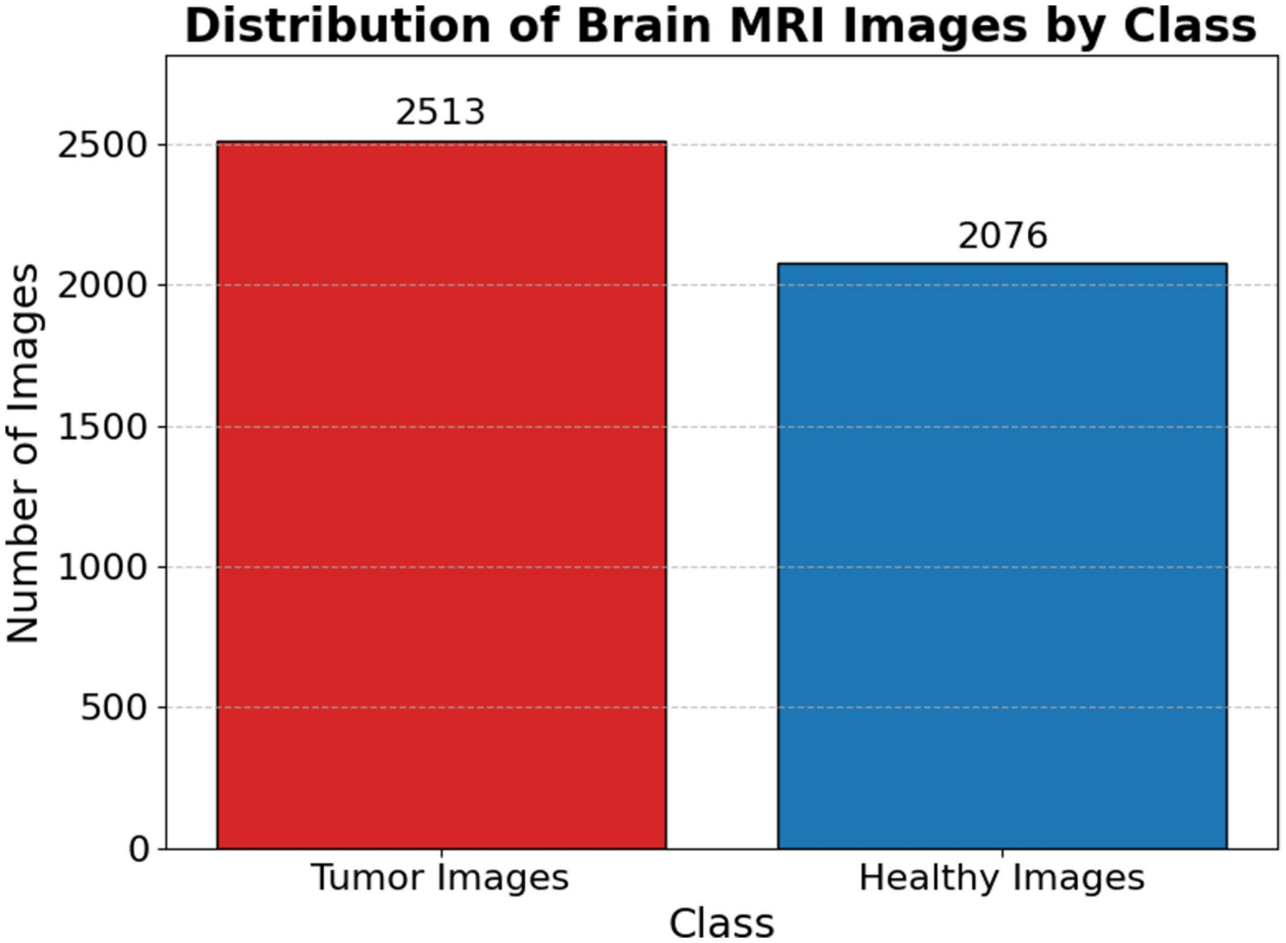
Distribution of brain MRI images scan by class.
